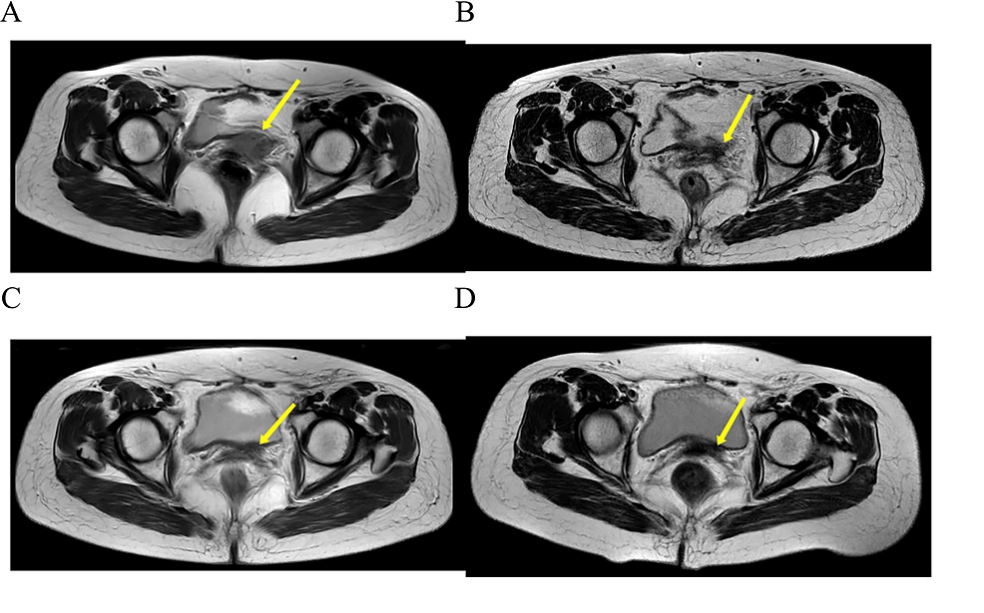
Enhanced magnetic resonance imaging of the pelvis A: Pre-treatment pelvic magnetic resonance imaging (MRI) shows a mass at the vaginal stump measuring approximately 3.0 x 2.2 cm (yellow arrow). B: Repeat pelvic MRI at three months after the end of treatment showed slight thickening of the vaginal stump. C: Repeat pelvic MRI at six months after the end of treatment showed slight thickening of the vaginal stump. D: Repeat pelvic MRI one year after the end of treatment showed slight thickening of the vaginal stump.
